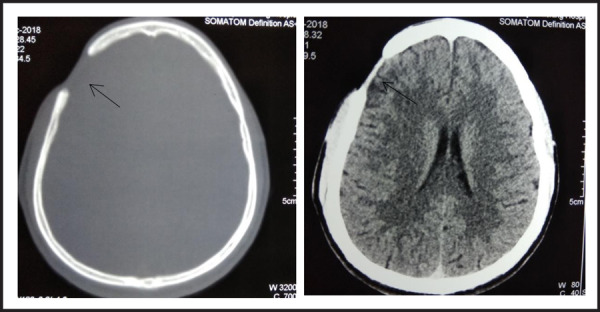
Plain Axial CT scan [(A) - Bone window (B) - Brain window] showing calvarial defect in right frontal bone without epidural and subgaleal collection.
